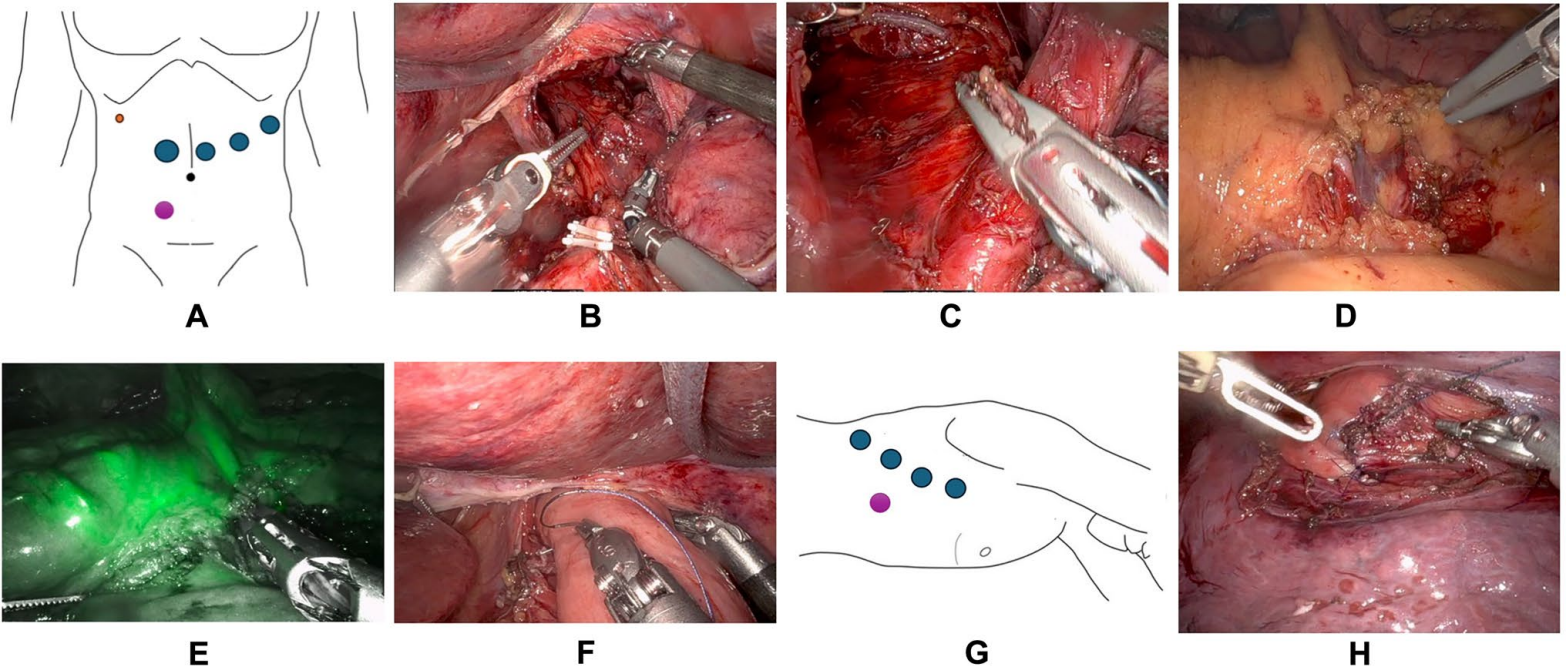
A Trocar positions for abdominal phase, B Circumferent esophageal mobilization high up in the mediastinum, C Transection of the esophagus high up in the mediastinum, D Isolation of jejunal vessels of the long roux limb, E Before division of jejunal vessels an ICG-control is performed with the vessels clamped to ensure adequate perfusion, F The long roux limb is brought up to the right pleura and sutured to the right crus, G Trocar positions for thoracic phase, H An end-to-side esophagojejunostomy is performed transthoracically
